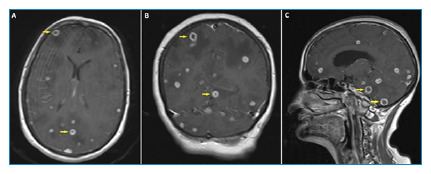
The axial (A), coronal (B), and sagittal (C) images of contrast-enhanced T1-weighted magnetic resonance imaging showing the foci of abscesses (tuberculomas) with peripheral enhancement.
